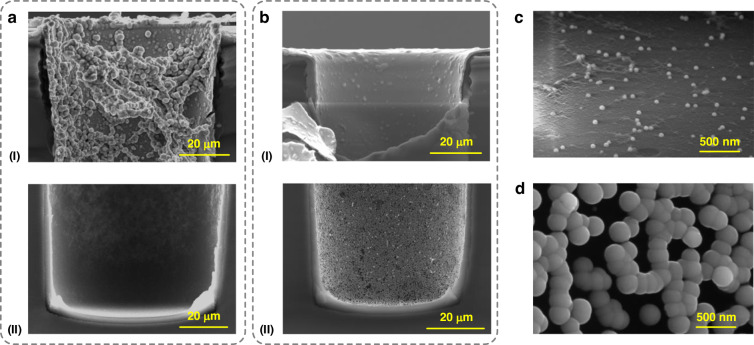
Deposition results of Cases A and B a SEM images of the Ni ELP results of Case A at the (I) via top and (II) via bottom. b Ni ELP results of Case B after performing ultrasound treatment with ultrasound power and pulse duty cycle values of 300 W and 1 s/10 s, respectively. c, d Magnified images of the via bottoms for Case A and B, respectively, showing the distributions and morphologies of the deposited Ni particles
